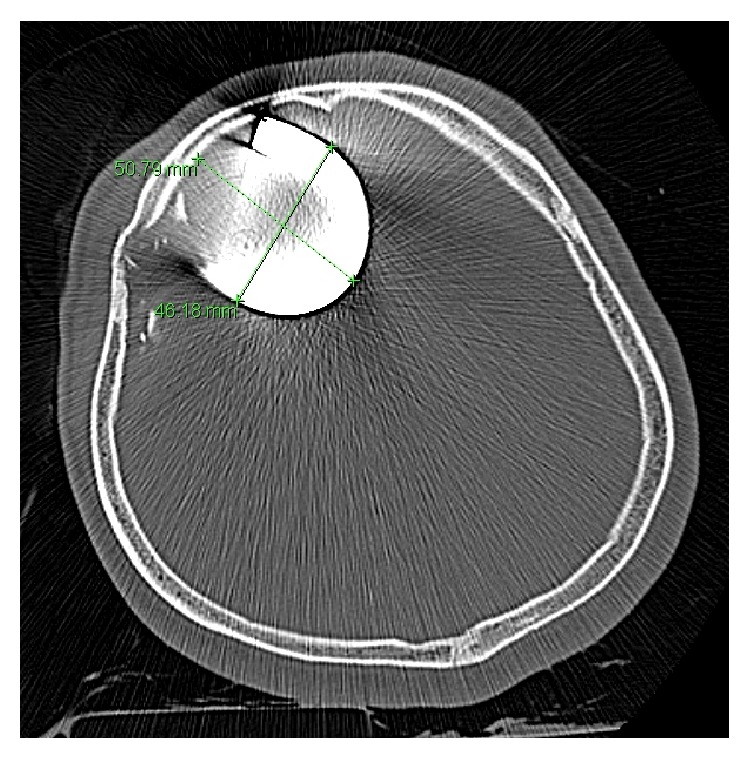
Axial computed tomography of the head (bone window), showing the intracranial penetration of a hook-shaped metal bar measuring 50.8 mm × 46.2 mm.
